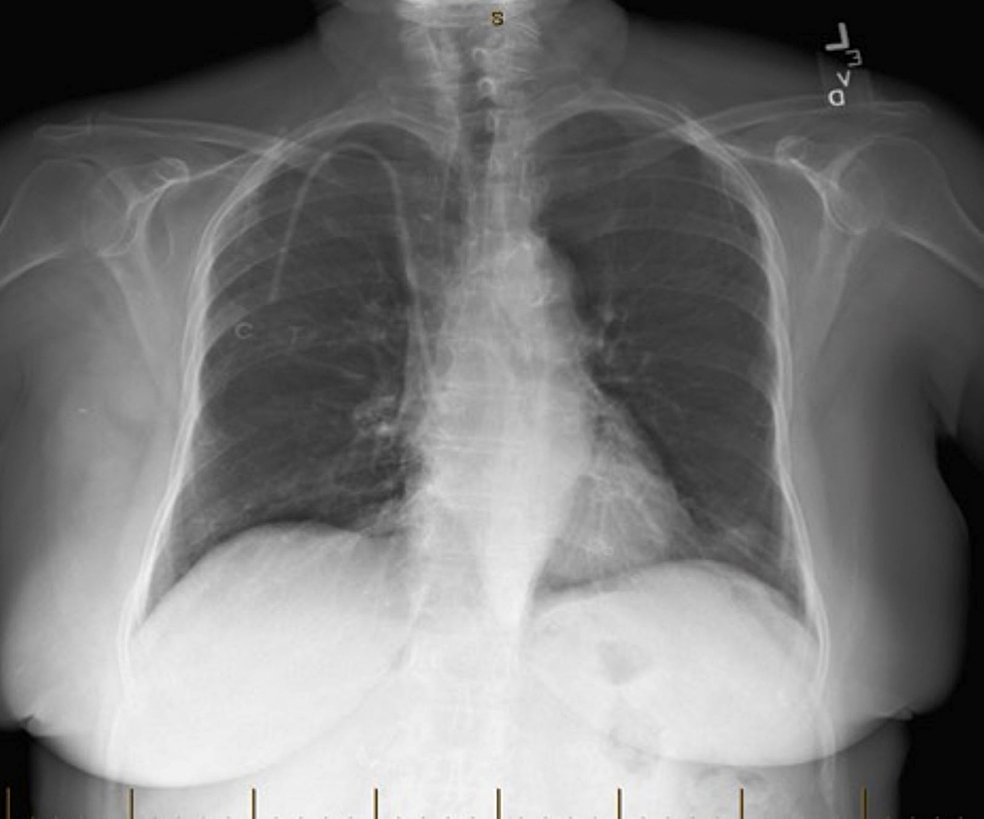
Portable chest X-ray taken 10 months prior to the day of admission that shows absence of the patchy infiltrates seen in Figure 2.
